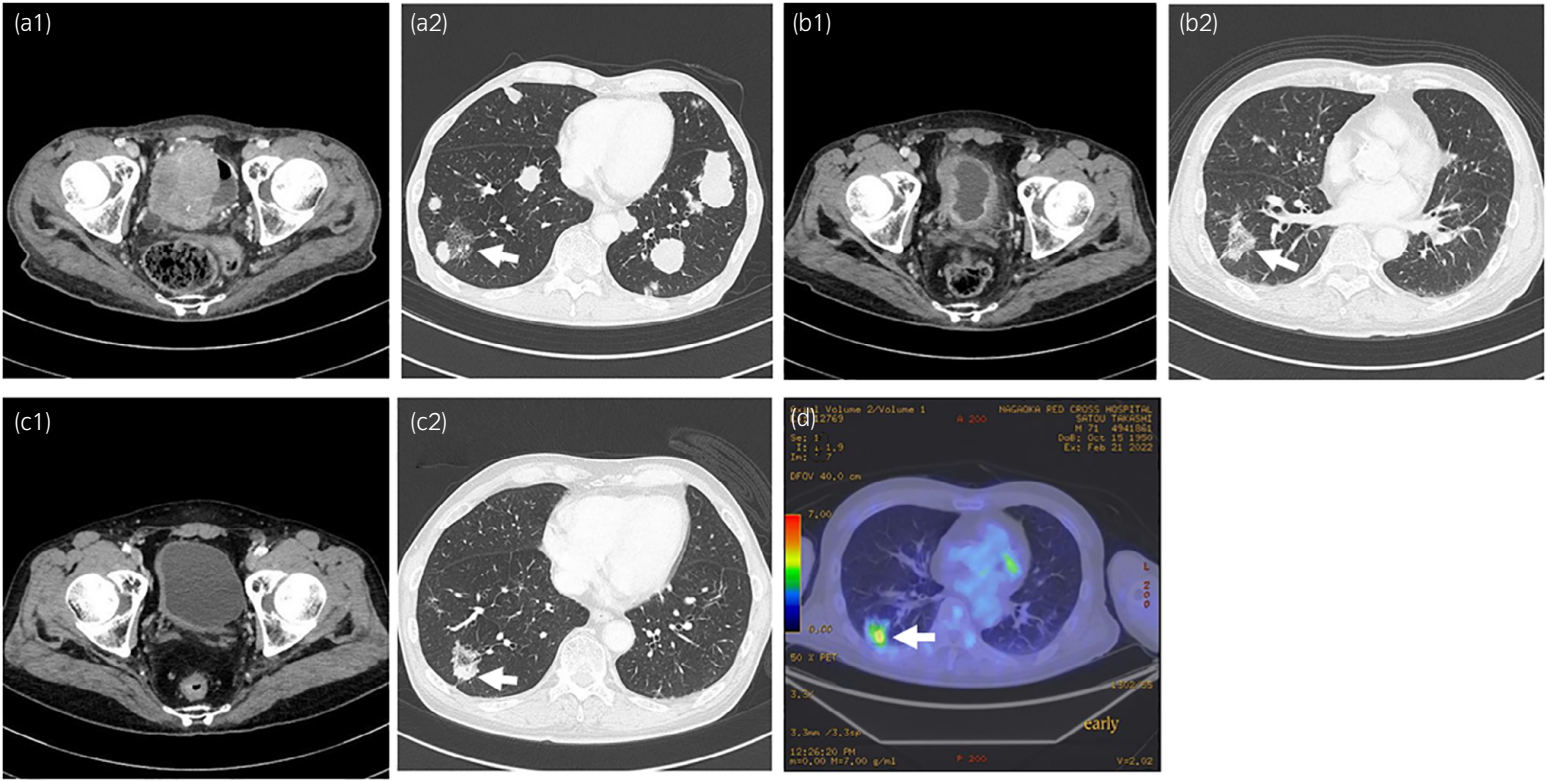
(a) CT at the time of admission showed large lesions in the bladder (a1), multiple lung metastases, and GGO lesion in the inferior lobe of the right lung (a2) (arrow). (b) CT after six courses of gemcitabine and carboplatin chemotherapy revealed decreases in the sizes of bladder cancer (b1) and multiple metastases (b2); however, the GGO lesion was observed in the lower lobe of the right lung (arrow). (c) CT in January 2022 revealed that decreases in the sizes of bladder cancer (c1) and multiple metastases (c2) were maintained during pembrolizumab therapy; however, the GGO lesion in the inferior lobe of the right lung gradually increased in size (arrow). (d) Fluorodeoxyglucose‐positron emission tomography showed the accumulation of fluorodeoxyglucose in the GGO lesion in the inferior lobe of the right lung (arrow).
